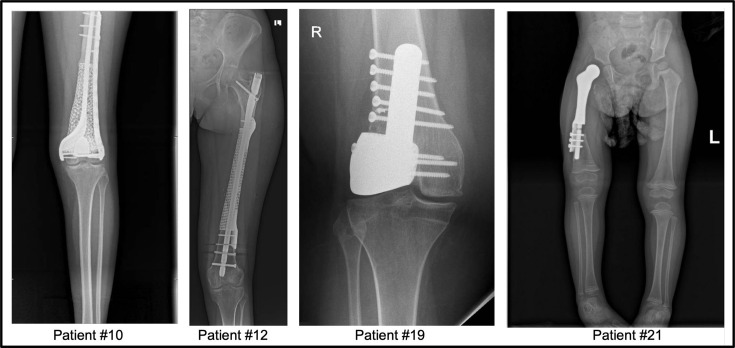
Anteroposterior postoperative radiographs demonstrating reconstruction with patient-specific Ti-6Al-4V lattice implants, from left to right showing: a) Patient #10, a 17-year-old female with distal femoral osteosarcoma reconstructed with a custom lattice implant and distal plate fixation. b) Patient #12, a 14-year-old female with mid-shaft femoral Ewing's sarcoma reconstructed with a custom lattice implant reinforced with an intramedullary nail. c) Patient #19, a 15-year-old male with distal femoral geographical chondroblastoma resection and reconstruction using a custom lattice implant. d) Patient #21, a two-year-old female with distal femoral osteosarcoma showing a custom proximal femur arthroplasty implant.
